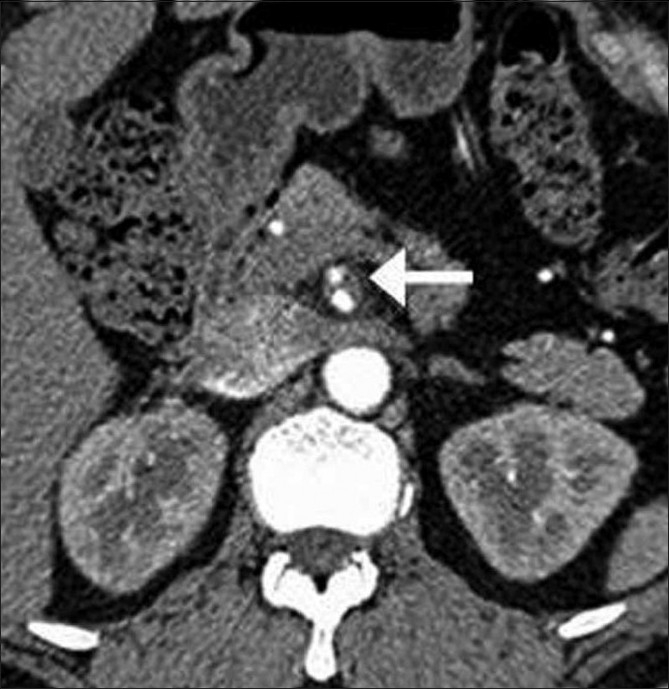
Axial, arterial-phase, contrast-enhanced, CT scan shows superior mesenteric artery dissection (arrow) with patent true and false lumens
